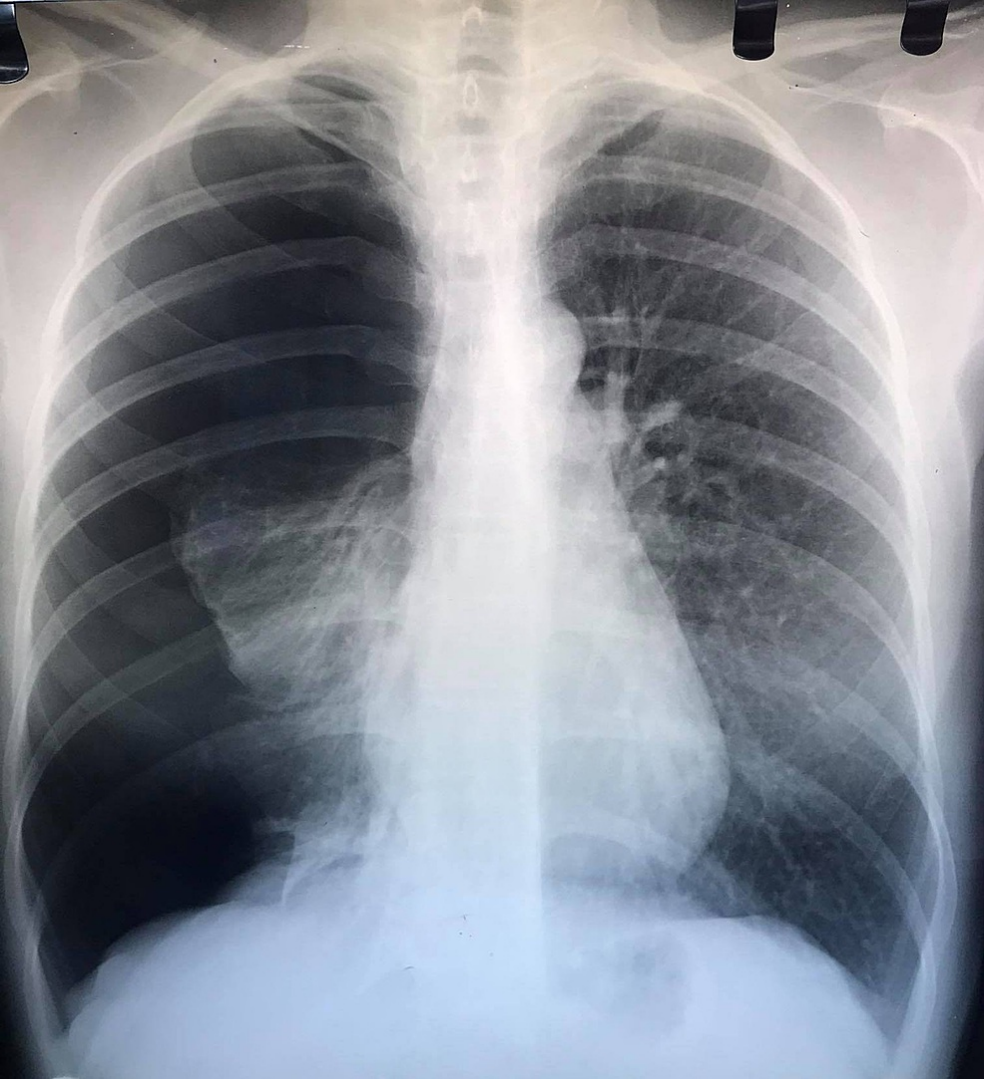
Chest X-ray showing a right pneumothorax with the right lung completely collapsed
